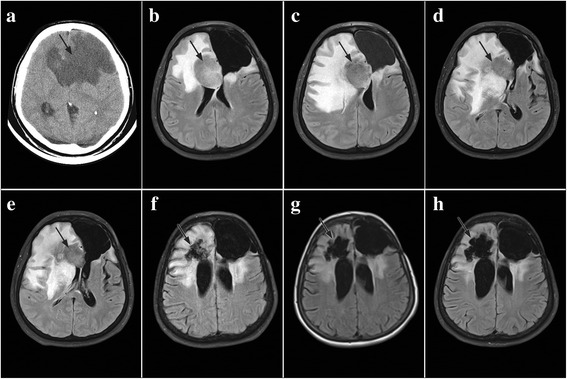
Overview of control scans of patient 4. Tumor tissue is indicated by arrows. a CT performed in March 2012, scan of tumor pre-surgery. b The NMRI from August 2012 shows the progression of the tumor, after which StPT was introduced. c The NMRI performed in November 2012 shows stopping of the progression after the introduction of StPT. d The NMRI from February 2013 shows a new progression of the tumor after which PTS was introduced. e The NMRI scans performed in May 2013 show regression of the tumor to 50 mm. f The control NMRI performed in October 2014 showed the further reduction of the tumor to 45 mm. g The NMRI performed in April 2015 showed that the tumor was approximately of the same dimensions as on the previous scan. h The scans performed in May 2016, 4 years after the initial diagnosis, showed that the tumor radius remained approximately of the same dimensions as on the previous two scans
